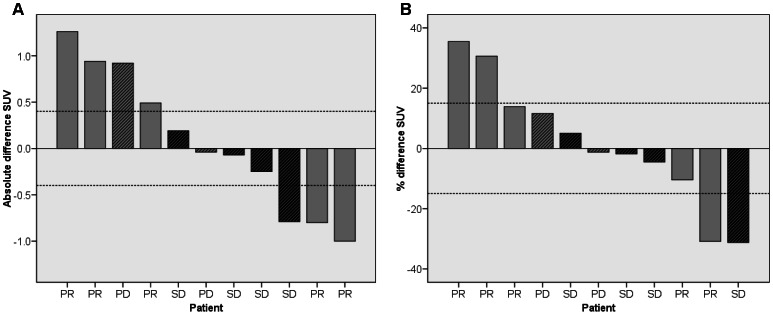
Change of ^18^F-FLT uptake. Change of ^18^F-FLT uptake in SUV 40–60 min normalised to bodyweight (A) absolute difference, (B) percentage difference.
